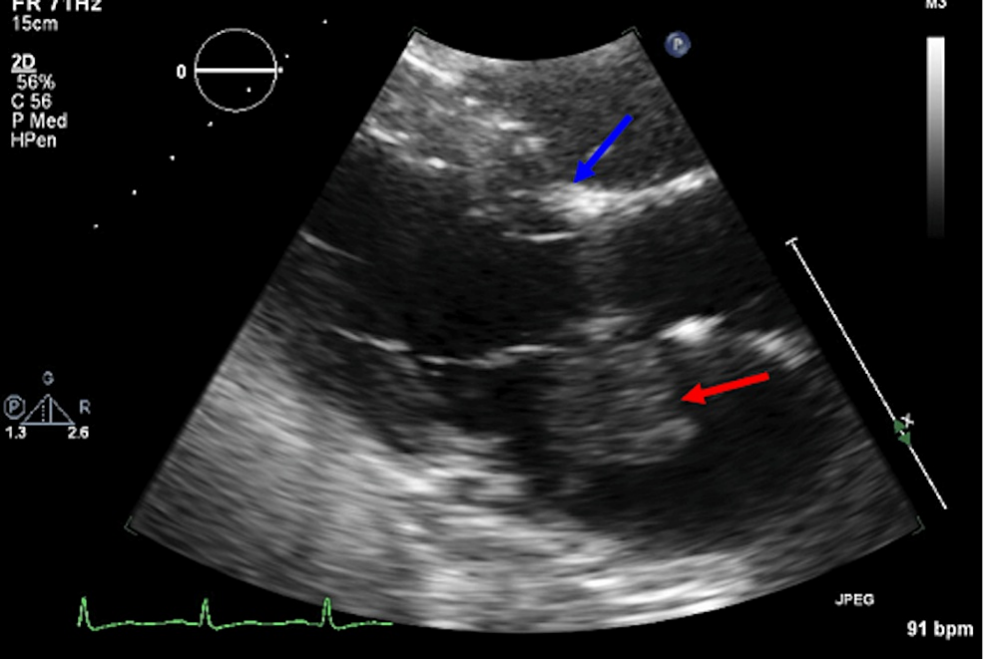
Echo identifies a large mass attached to the atrial septum (blue arrows), measuring 3.4x3.4 cm, very mobile, and entering the left ventricle with each diastolic cycle (red arrow). Echo: echocardiography
